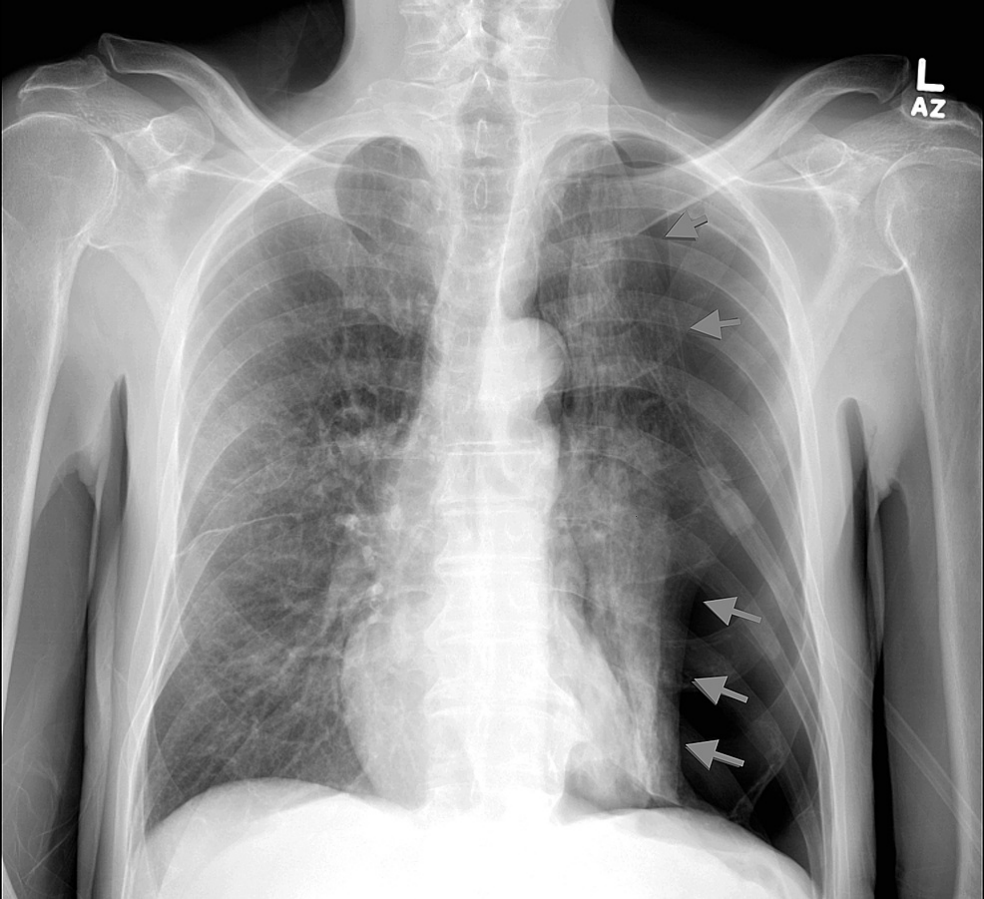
CXR with delayed PTX post-biopsy (arrows) CXR: chest radiograph; PTX: pneumothorax
